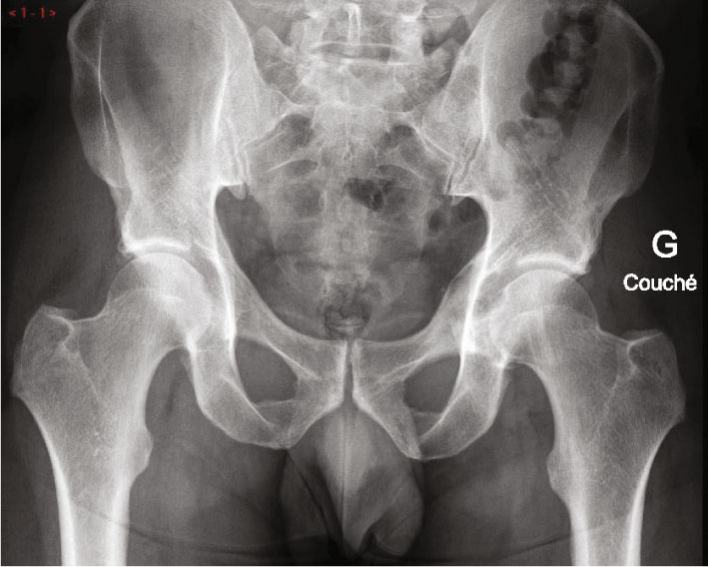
AP pelvic plain film showing a lytic lesion of the left iliopubic ramus.
